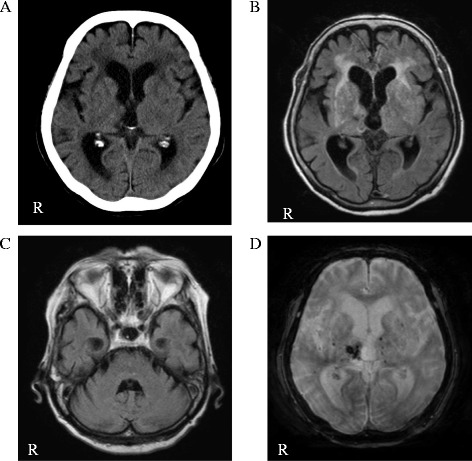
a. Brain computed tomography did not show basal ganglia calcification. b. Magnetic resonance imaging fluid-attenuated inversion recovery showed slit hyperintensity of the lateral margin of the right putamen but did not show appreciable atrophy of the basal ganglia. c. Magnetic resonance imaging fluid-attenuated inversion recovery did not show appreciable atrophy of the pons and cerebellum. d. T2* magnetic resonance imaging showed an old intracranial hemorrhage in the right thalamus. “R” indicates the right side
